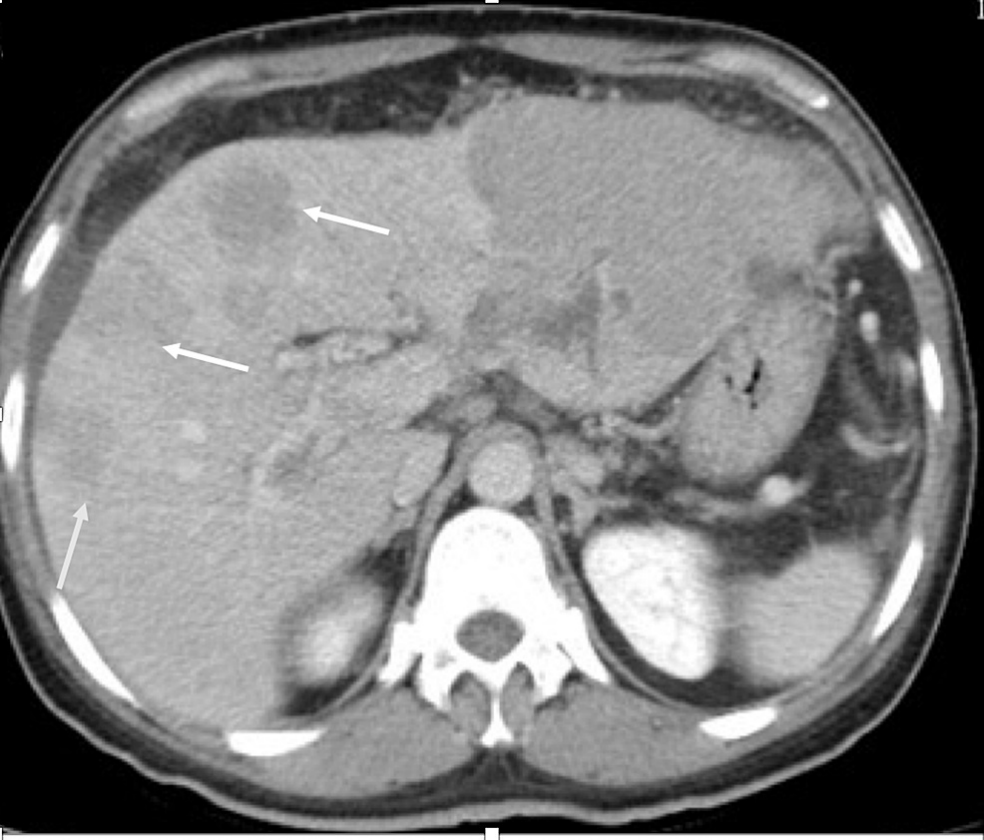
Abdomen CT scan: axial view White arrow: Multiple hypervascular tumors measuring up to 6 cm in size
